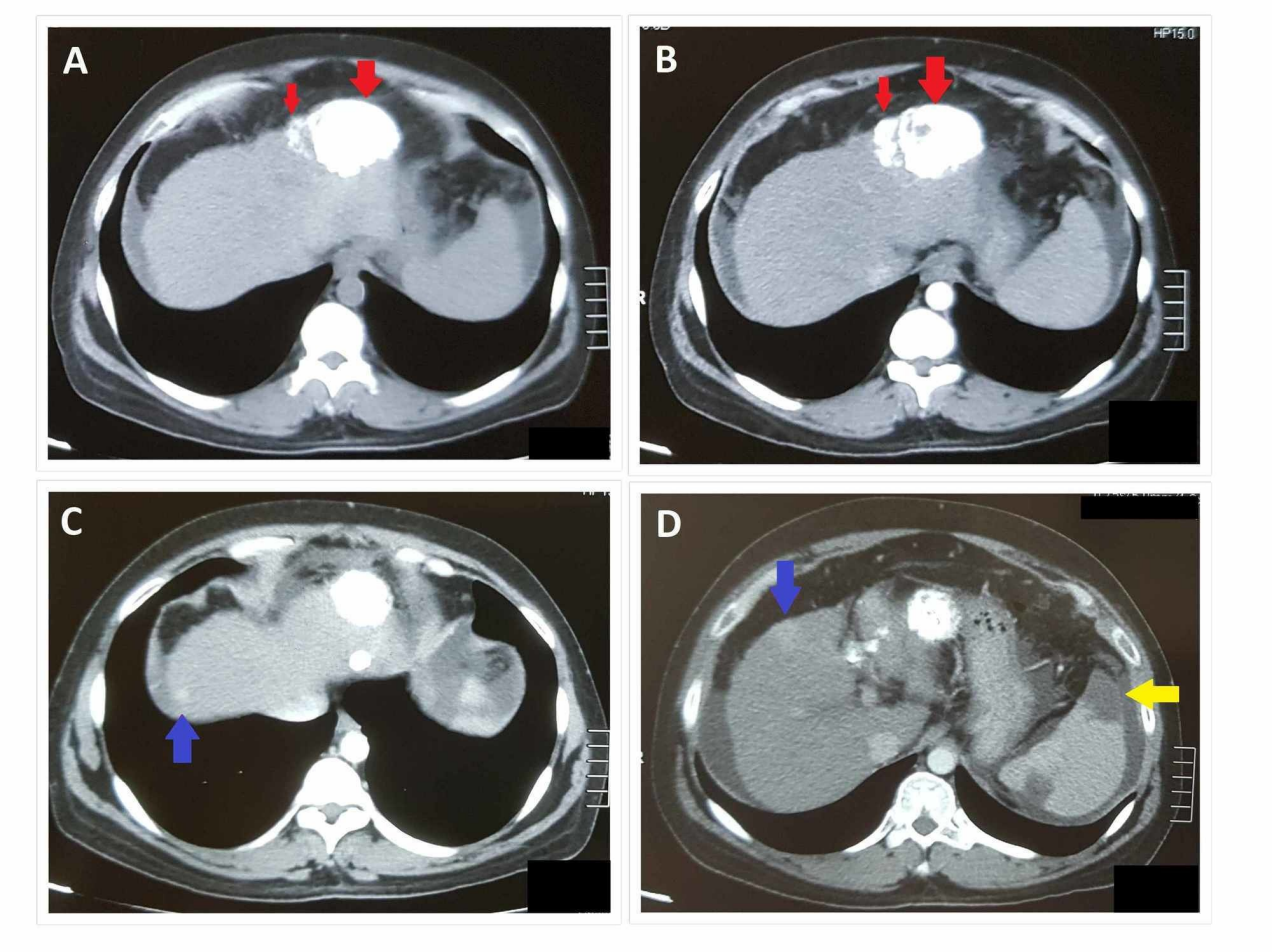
Triphasic CT abdomen at one-month follow-up after TACE showing two areas of dense hyperdensities (red arrows in A and B) in the left lobe of the liver at site of previous HCC representing lipoidal deposition (status post TACE) There is a new development of two small arterially enhancing lesions in the right lobe of the liver (blue arrows in C and D), suggestive of hepatomas. Spleen appears mildly enlarged. Wedge-shaped peripheral hypodense areas (yellow arrow) are seen within the splenic parenchyma suggestive of splenic infarcts. CT: computed tomography; TACE: transarterial chemoembolization; HCC: hepatocellular carcinoma
